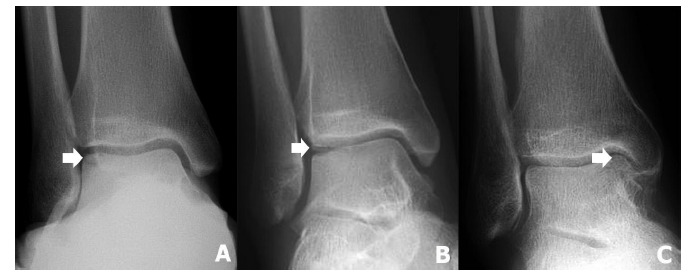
The radiography demonstrates the osteochondral lesions of the talus (OLT). The anterolateral lesions of OLT, Berndt and Harty type 2, 3, and 4, are demonstrated on Figs
